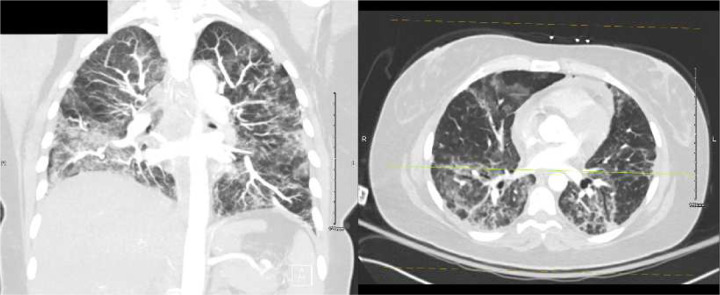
Outpatient computed tomography one day before second admissions, showing worsening multifocal pneumonia.
